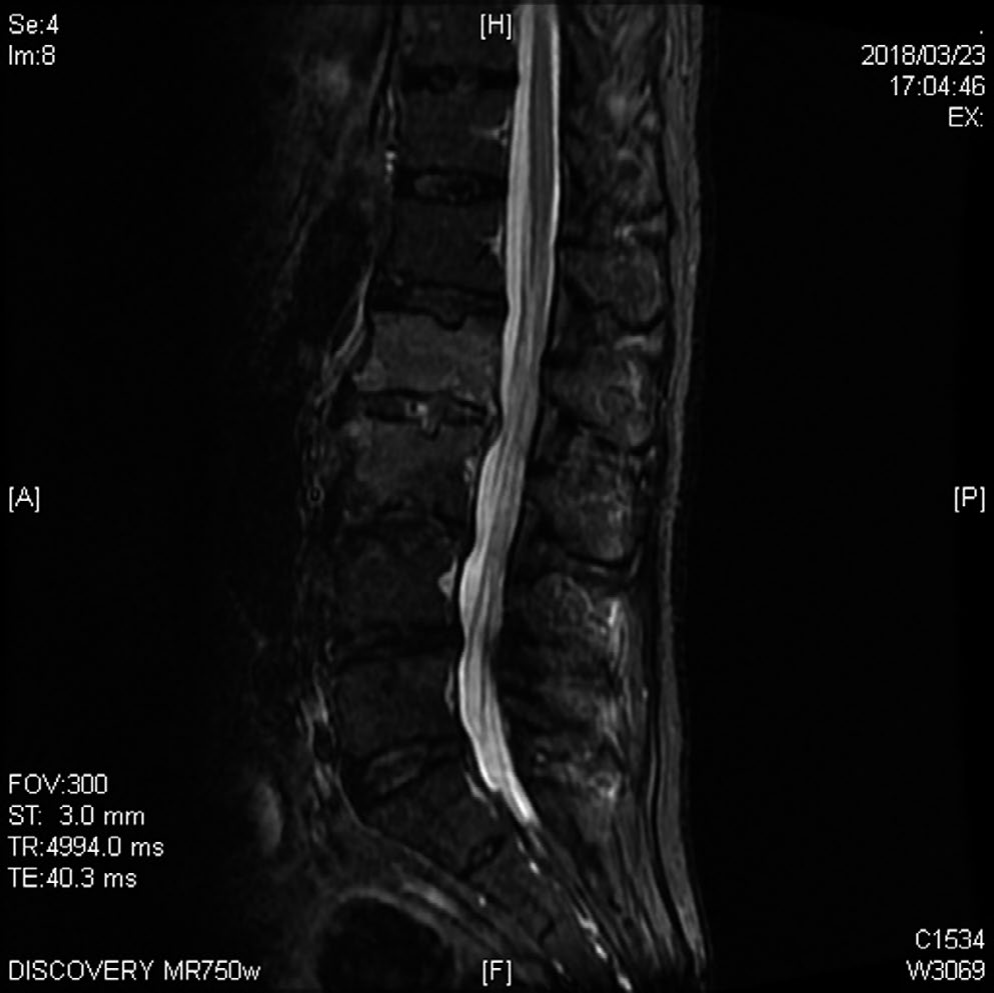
An MRI imaging of lumbar vertebrae on day 17. High intensity on STIR images was seen on the first and second vertebrae
